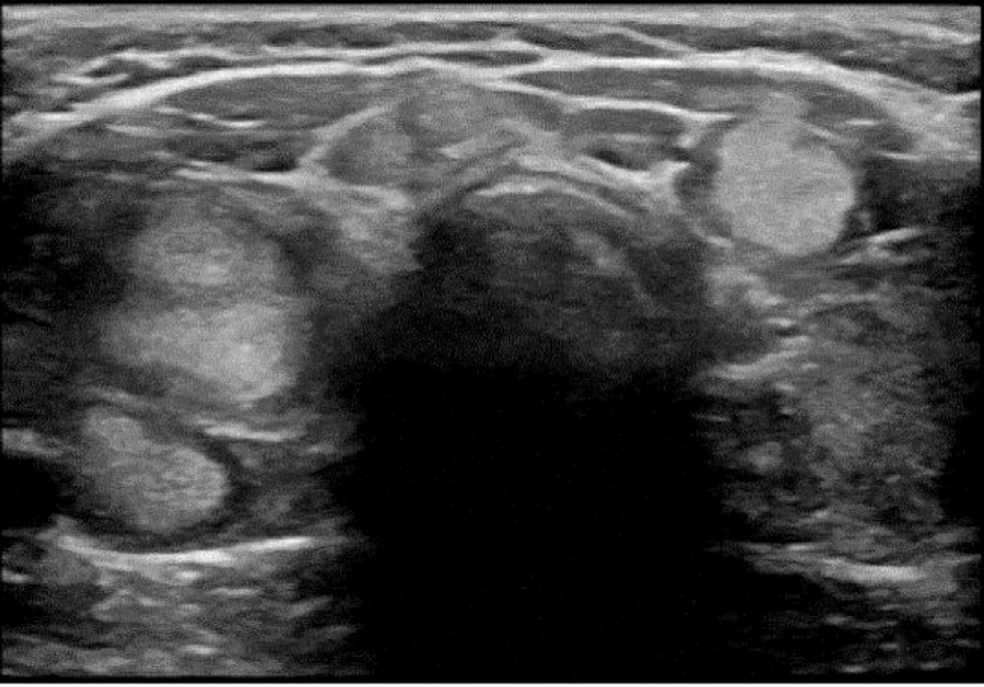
Thyroid ultrasound of Case 1 showing multinodular goiter with nodules size ranging from 1 to 2.3 cm.
